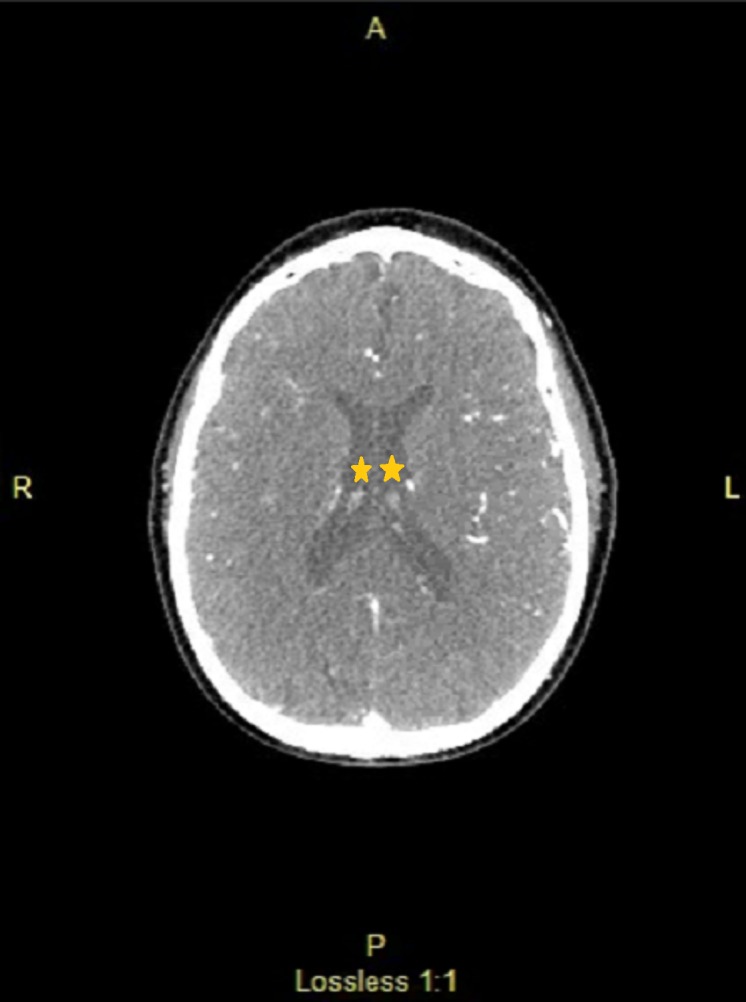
Computed tomography (CT) head showing no acute bleeding and no midline shift. Ventricles marked by star showing absence of bleeding or midline shift.
